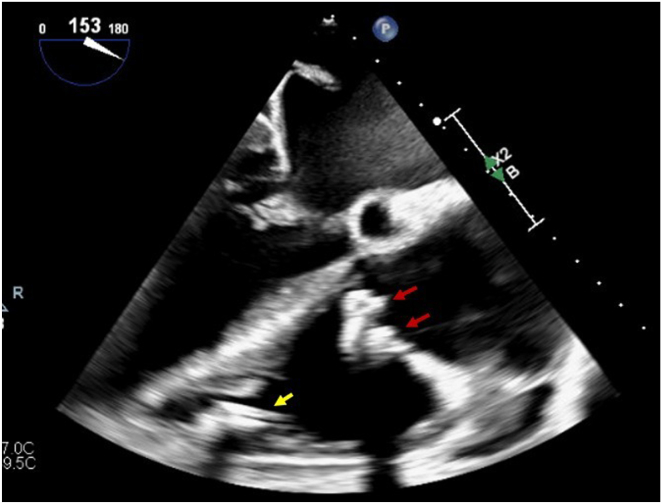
TEE mid-esophageal view at 153 degrees. Red arrows showed 2 TV clips, yellow arrow shows Micra VR. TEE = t*ransesophageal echocardiography*; TV = tricuspid valve.
